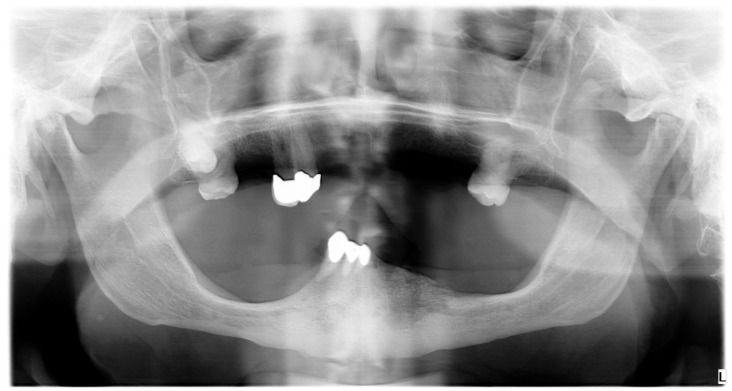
Panoramic Radiogram. The panoramic radiogram shows the osteolytic area overlapping with the lower left mandibular canal.
